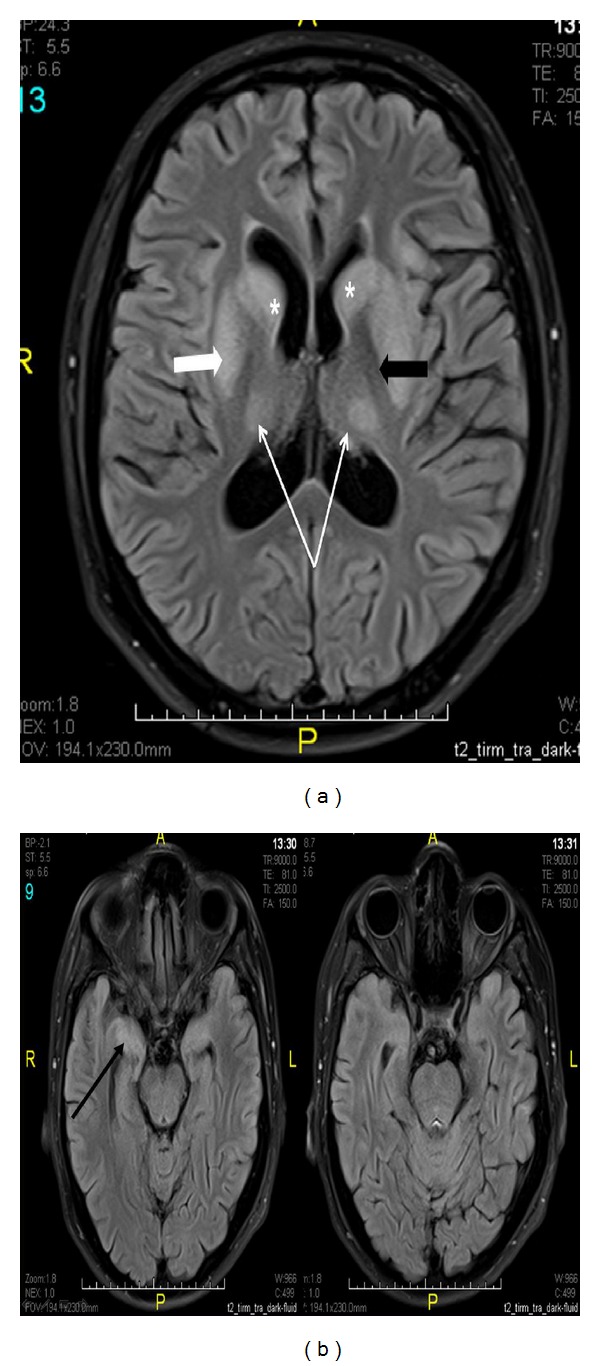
(a) MRI brain: axial FLAIR image showing hyperintense signal in the caudate nuclei bilaterally (asterisk marks), both putamina bilaterally (white thick arrow pointing to the right putamen), and both thalami (thin white arrows). Note the sparring of the globus pallidi (black thick arrow). (b) Axial FLAIR of the brain showing increased signal in the temporal lobes (namely, the anterior temporal lobes, particularly the hippocampi (black arrow)). The Pons show no sign of demyelination.
